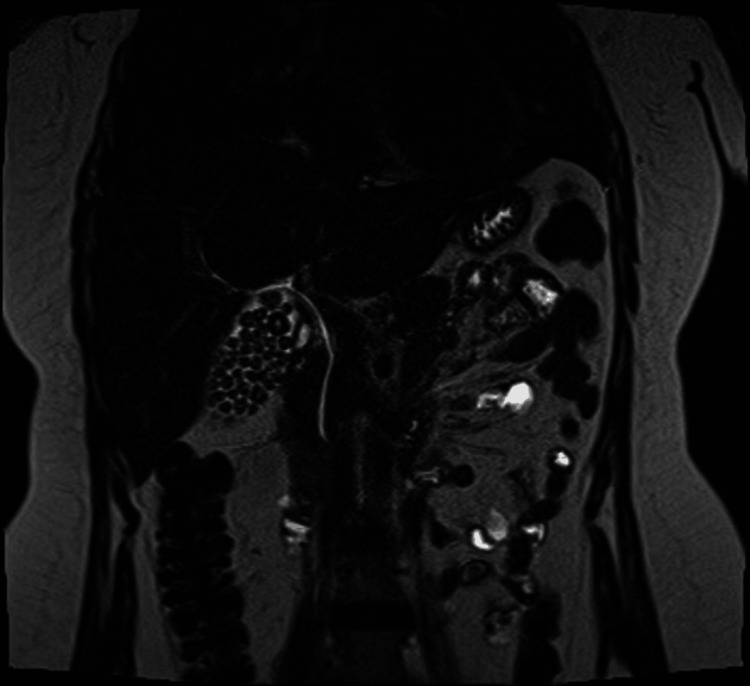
MRI: coronal plane The gallbladder is distended, measuring 9.7 cm in its longest axis, with numerous faceted calculi in its lumen ranging in size from 5 to 11 mm. The cystic duct exhibits normal caliber and insertion. The intrahepatic bile ducts display normal caliber and signal intensity. The extrahepatic bile duct measures 4 mm in internal diameter at the level of the common hepatic duct. No hypointense filling defects suggestive of choledocholithiasis are observed in the common bile duct MRI: magnetic resonance imaging
